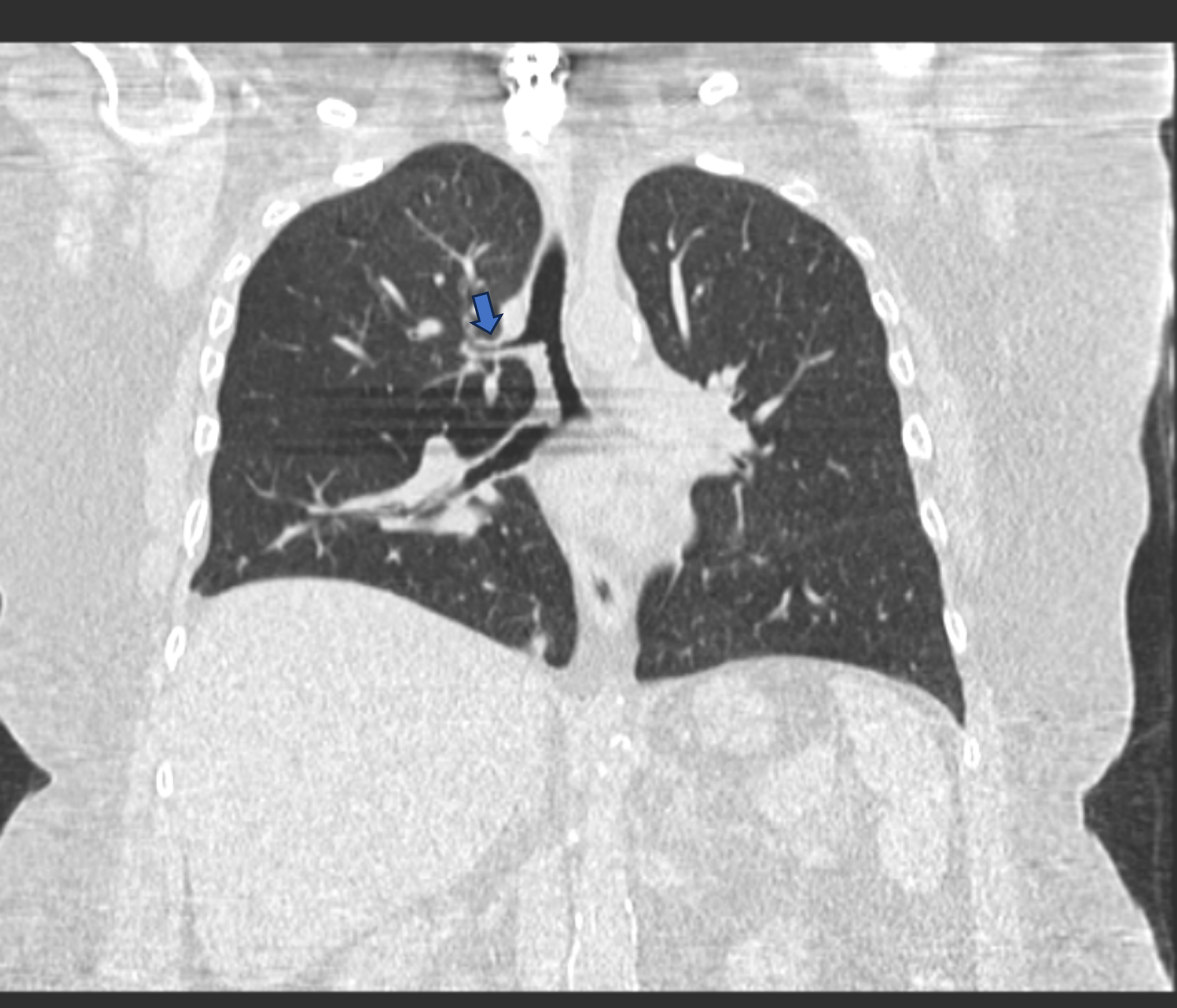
Computed tomography chest, arrow mark indicating the right tracheal bronchus.
